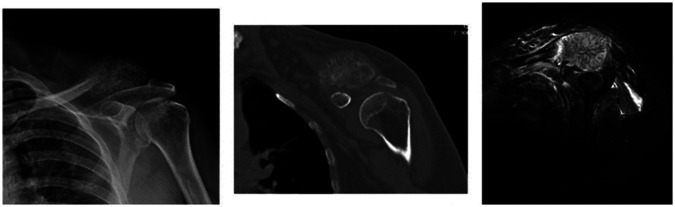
Preoperative images of the patient: direct radiograph, CT, and MRI.
